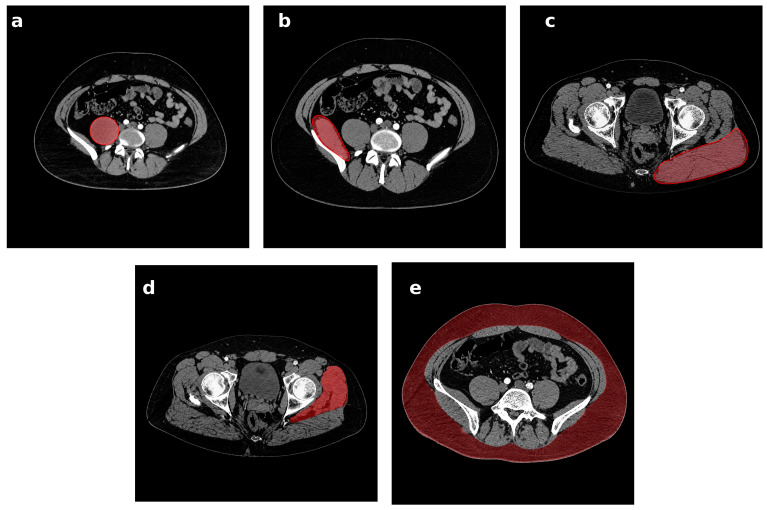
Representative axial computed tomography images showing the standardised anatomical levels at which pelvic body-composition measurements were obtained on the contralateral (uninjured) hemipelvis. The segmented region of interest for each structure is shaded in red. (a) Psoas major at the L4–L5 intervertebral level; (b) iliacus at the L4–L5 intervertebral level; (c) gluteus maximus at the level of the centre of the acetabulum; (d) gluteus medius–minimus at the level of the centre of the acetabulum; (e) subcutaneous adipose tissue at the L5 vertebral body level.
